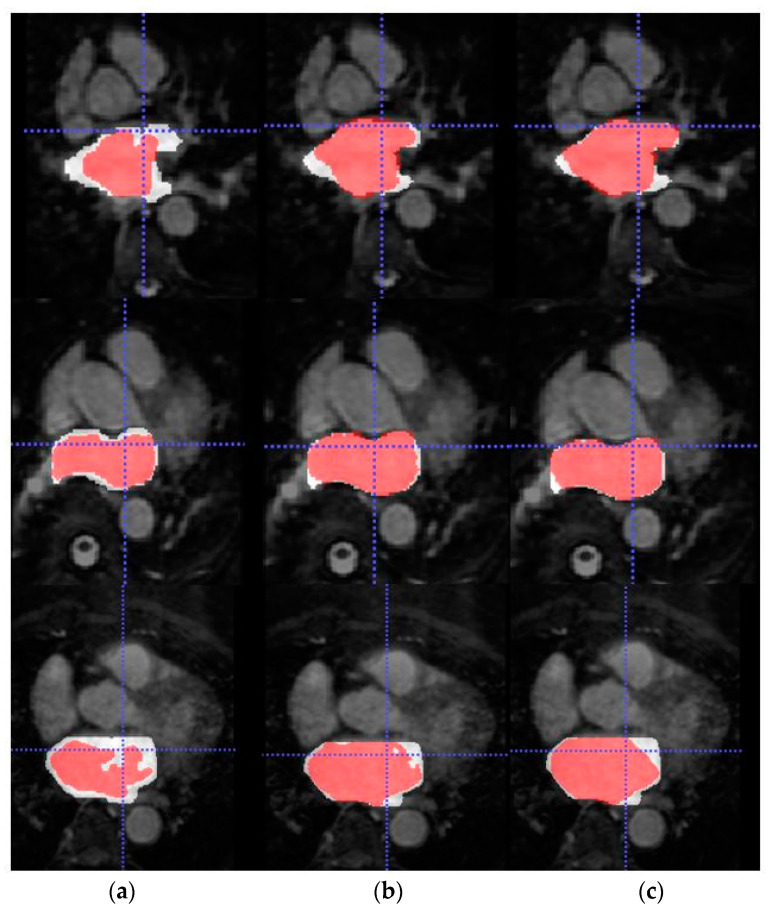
From top to bottom, axial slice of LA segmentations for three patients obtained through models (a) LGE-MRI, (b) w/o SDA and (c) w-SDA. Ground-truth mask in white, prediction mask in red and intersection in pink.
